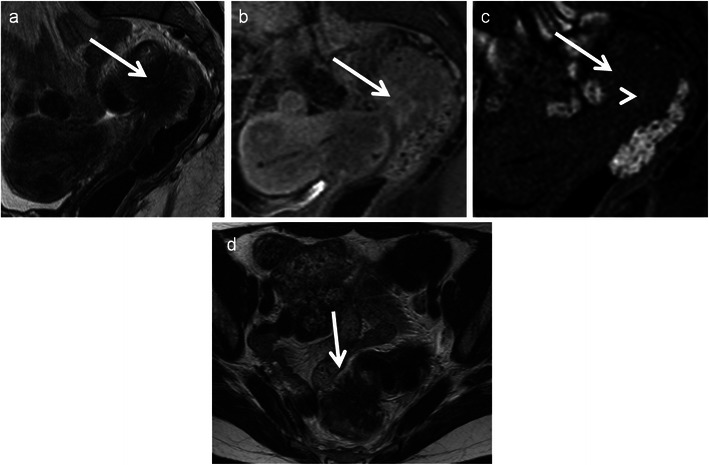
Rectal endometriosis. a Sagittal T2-weighted MRI reveals a hypointense spiculated irregular mass (*arrow*) along the anterior surface of the mid rectum. b Sagittal T1-weighted MRI reveals this fibrous deposit to be intermediate signal intensity. c Sagittal fat-suppressed T1-weighted MRI demonstrates tiny hyperintense foci (*arrowhead*) within the endometriotic deposit (*arrow*). d Axial T2-weighted MRI reveals the irregular spiculated hypointense lesion (*arrow*) with a few tiny hyperintense foci
